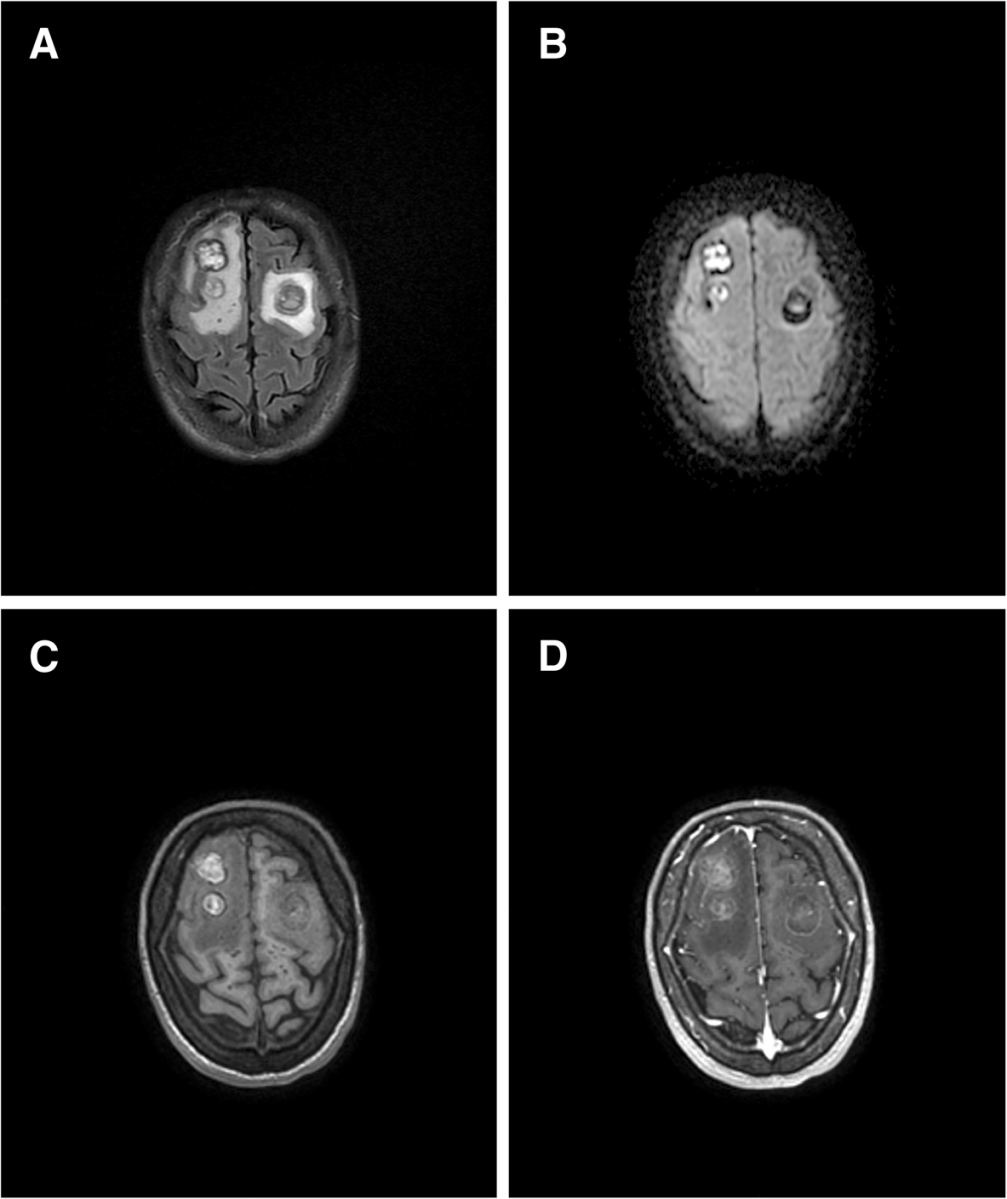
Magnetic Resonance Images (MRI) of the brain at control after 26 days of anti-*Toxoplasma *treatment. A. On Axial FLAIR T2-weighted images the lesions are hyperintense, with hypointense halo and surrounding edema. B. Diffusion-weighted imaging of the lesions showed restricted diffusion. C. On unenhanced Axial T1-weighted images the lesions signal is heterogeneous. D. On enhanced Axial T1-weighted images faint lesions enhancement are depicted.
